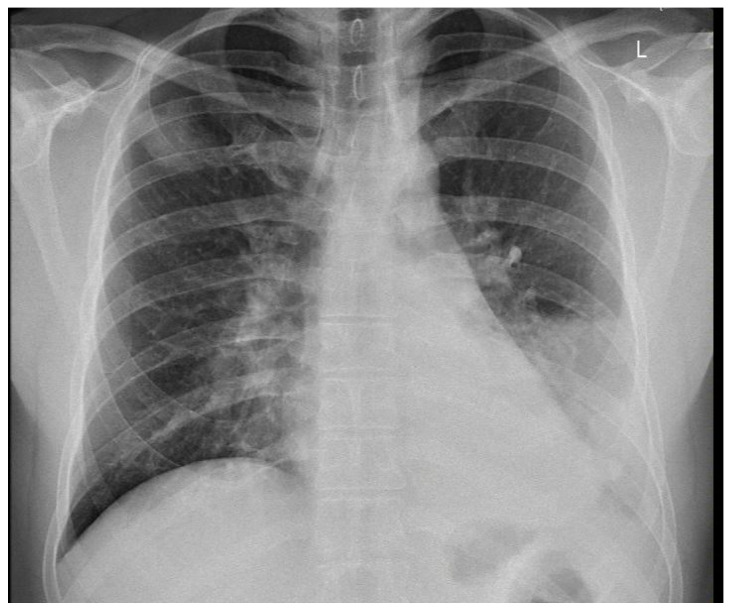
Left lung lower lobe infiltrates are shown, as well as a small amount of fluid in the left pleural cavity at X-ray scan.
